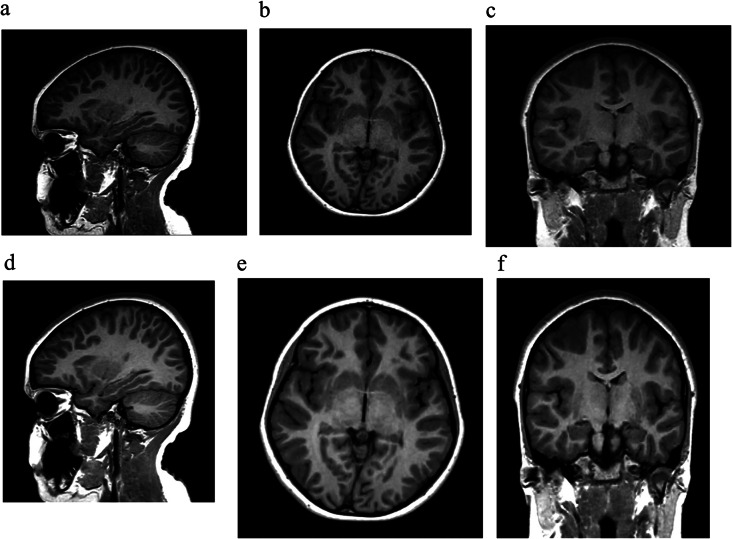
(a-f): Sagittal 3D T1-weighted SPGR CS = 2.5 with axial and coronal reformats from the clinical protocol was performed with standard (a-c) and AI (d-f) reconstruction, with little perceived difference in overall image quality
